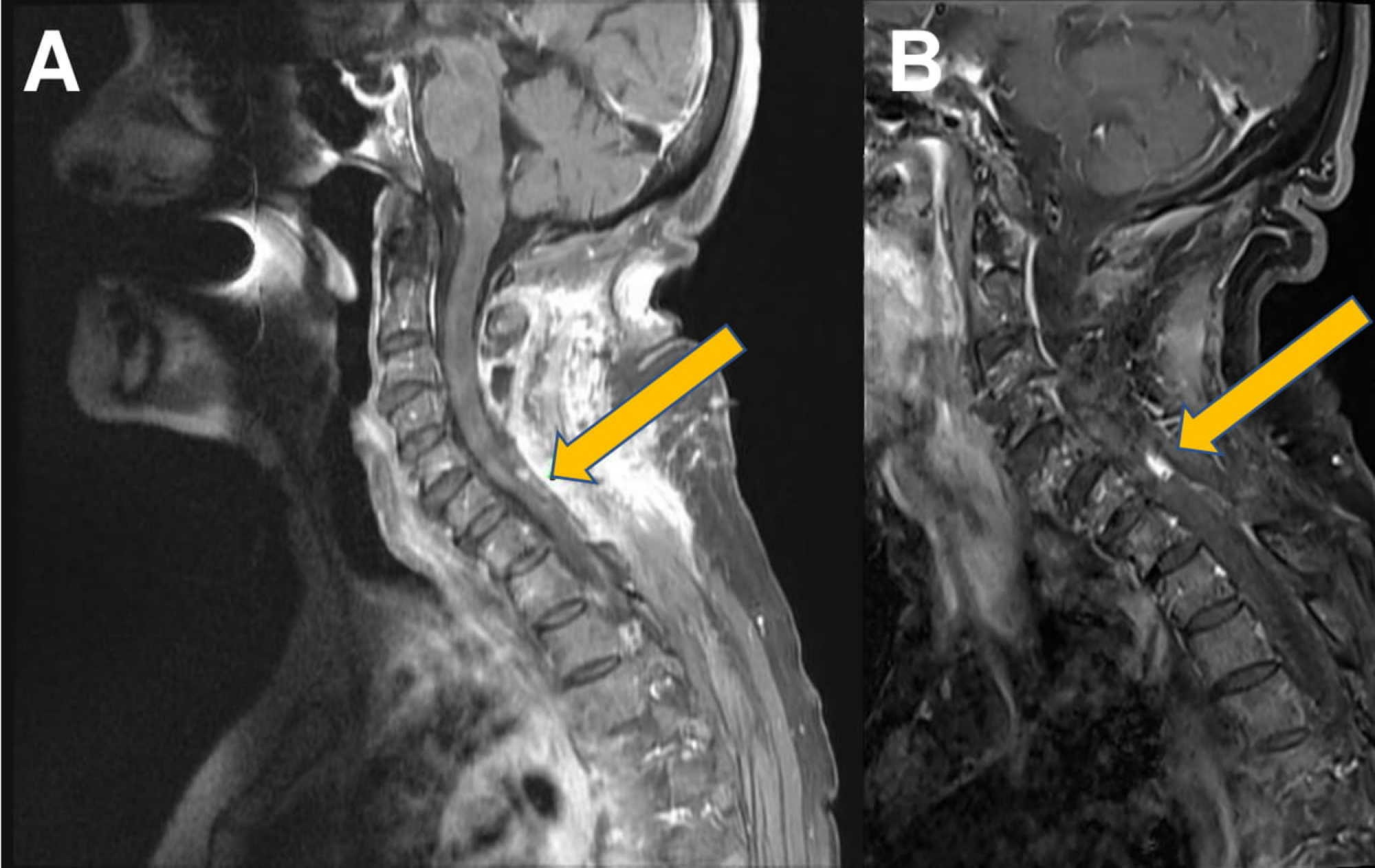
Postoperative nodule and lack of major postoperative growth (A) Fluid-attenuated inversion recovery magnetic resonance imaging (FLAIR MRI) with contrast (four and a half years after initial operation, two months after second operation); (B) FLAIR MRI with contrast (13.5 years after initial operation, nine years after second operation)
